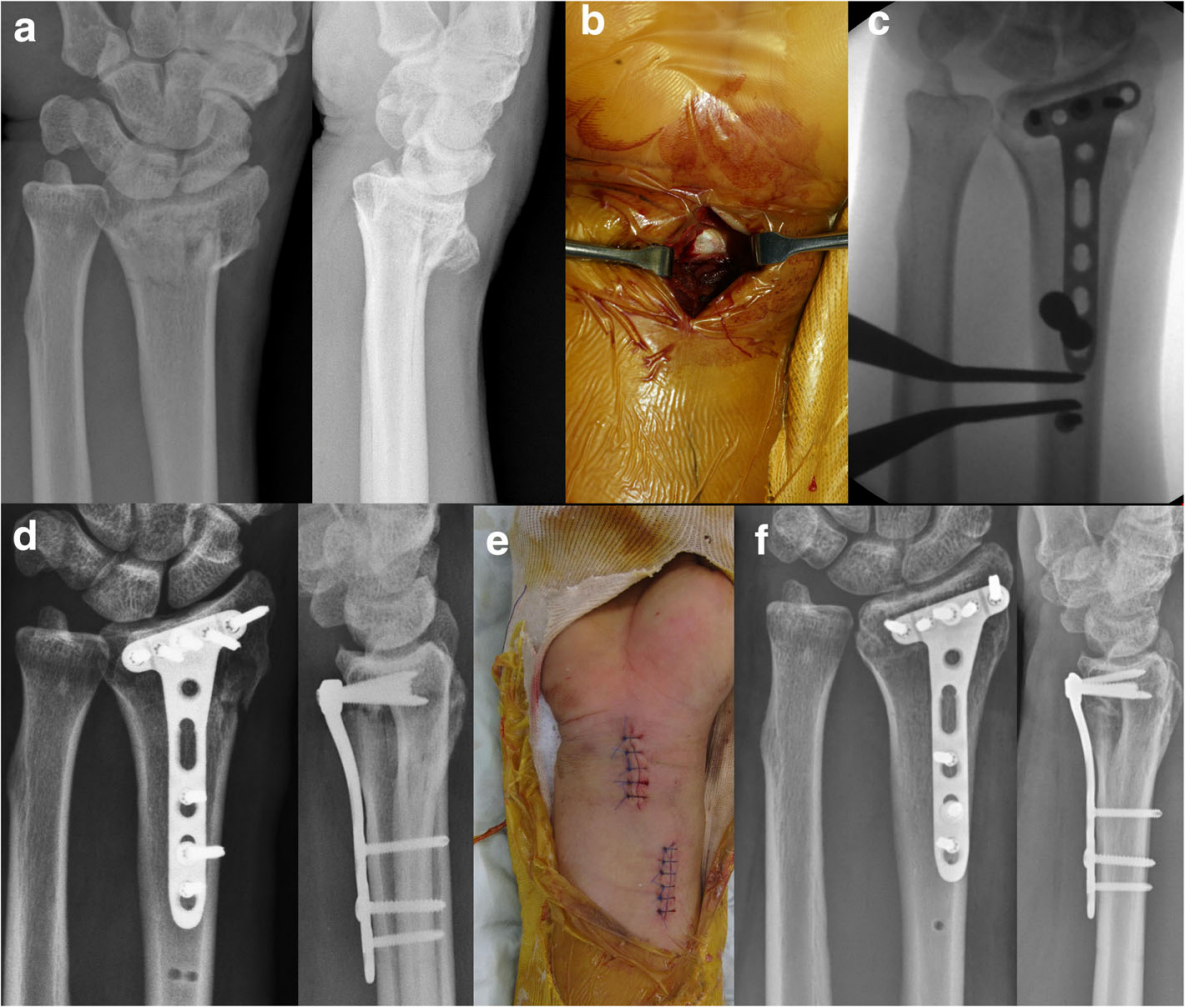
a Simple radiographs, showing a distal radial fracture with metaphyseal comminution and radial shortening. b A distal incision around the flexor carpi radialis tendon, exposing the distal margin of the PQ muscle. c Sliding of the plate through a PQ-sparing approach and the distractive technique to restore radial length using the spreader. d Postoperative radiographs showing good realignment of the distal radial fracture. e Two separated incisions. f After 16 weeks, the distal radial fracture was well healed without radial length shortening
